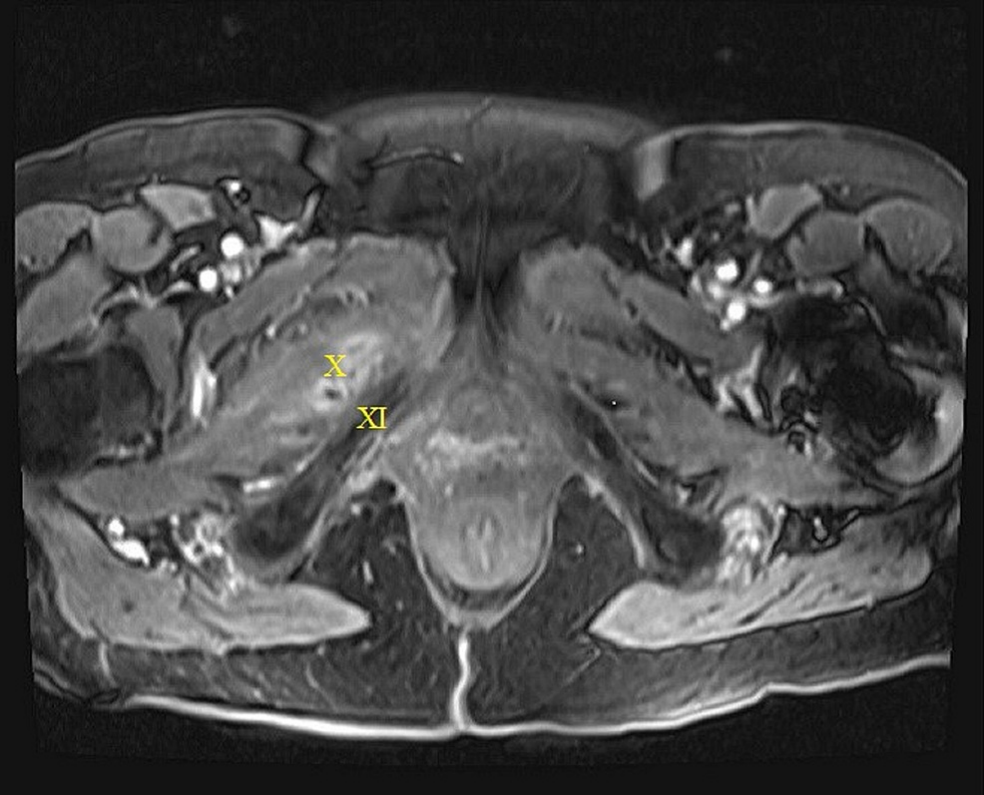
MRI taken on May 2018. Vaginocutaneous fistula tracking around the pubic ramus through the right anterior pelvic and thigh musculature and terminating at the surface of the right medial thigh lateral to the right labia X = fistula; XI = pubic ramus
